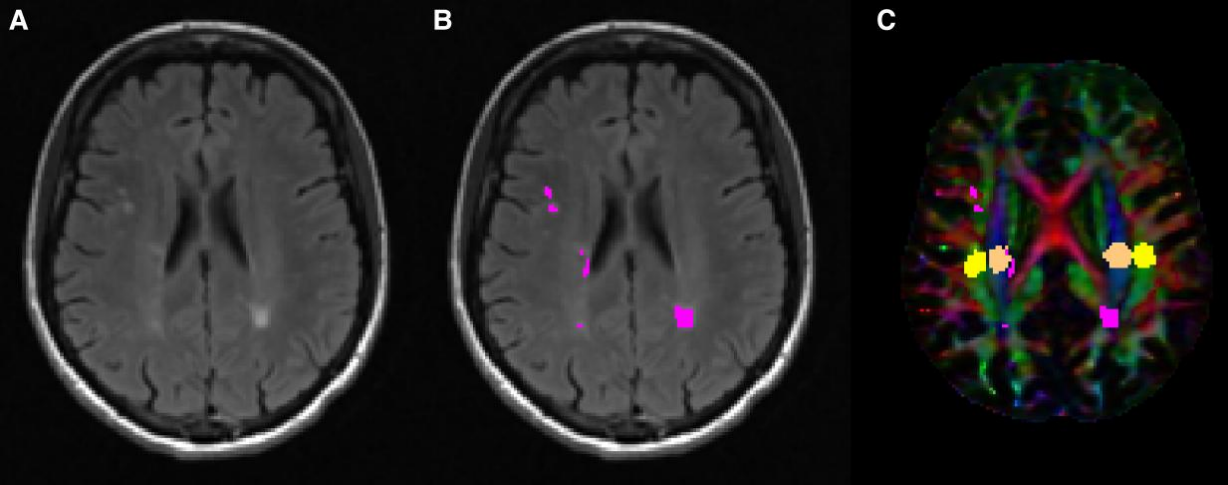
Representative images from a person with multiple sclerosis. (A) shows the T2-weighted fluid-attenuated inversion recovery (FLAIR) image from a representative person with multiple sclerosis. (B) shows the same T2-weighted FLAIR image with segmented T2 lesions overlaid in pink. (C) shows the color-coded primary vector modulated by the corresponding fractional anisotropy image. Lesions are again shown in pink while the SLF (association fibre) is shown in yellow and the corona radiata (projection fibre) is shown in orange.
